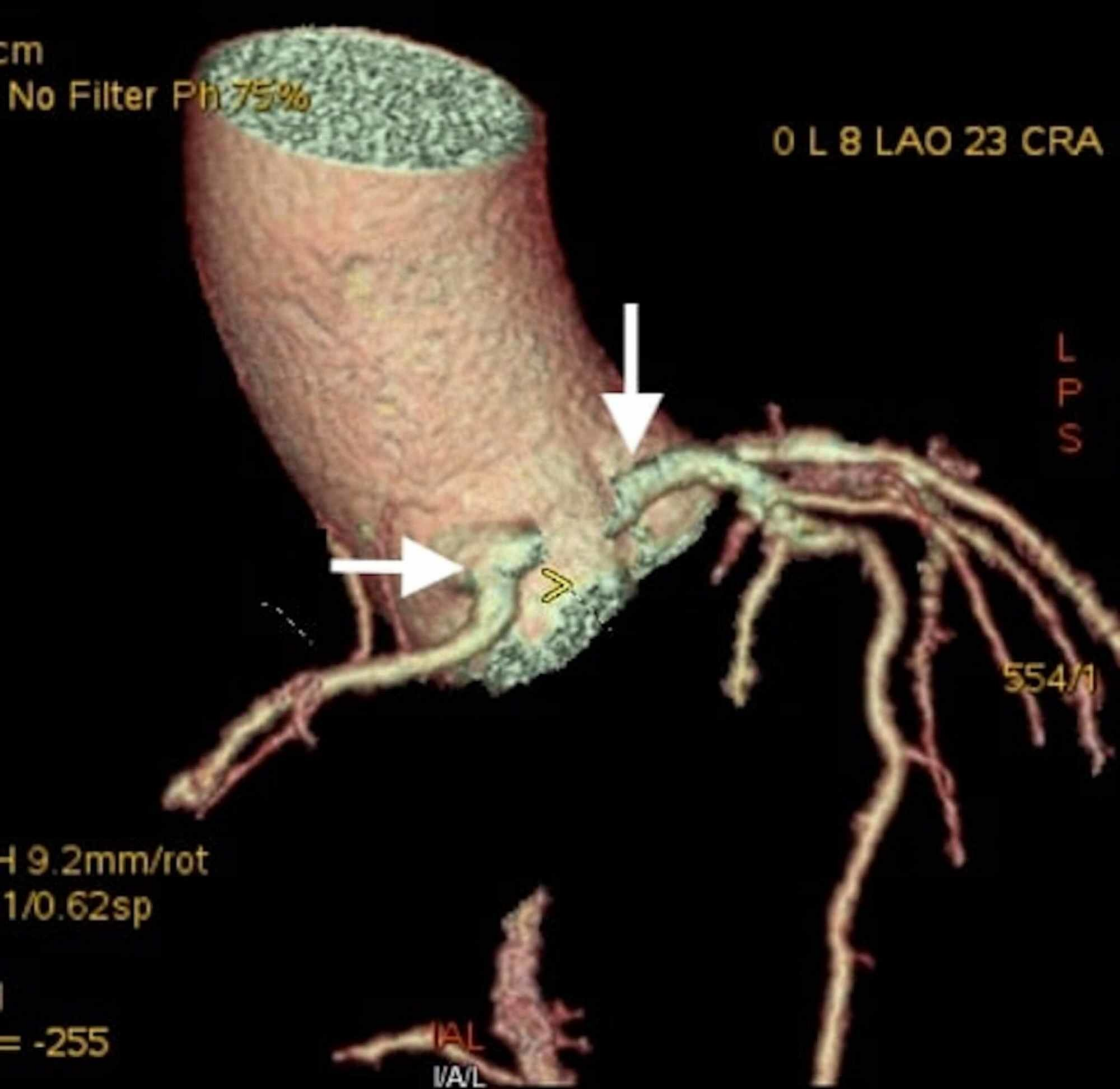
Coronary CTA image showing the RCA (horizontal white arrow) and LMCA (vertical white arrow) originating from the right coronary cusp CTA - computerized tomography angiogram; RCA - right coronary cusp; LMCA - left main coronary artery
